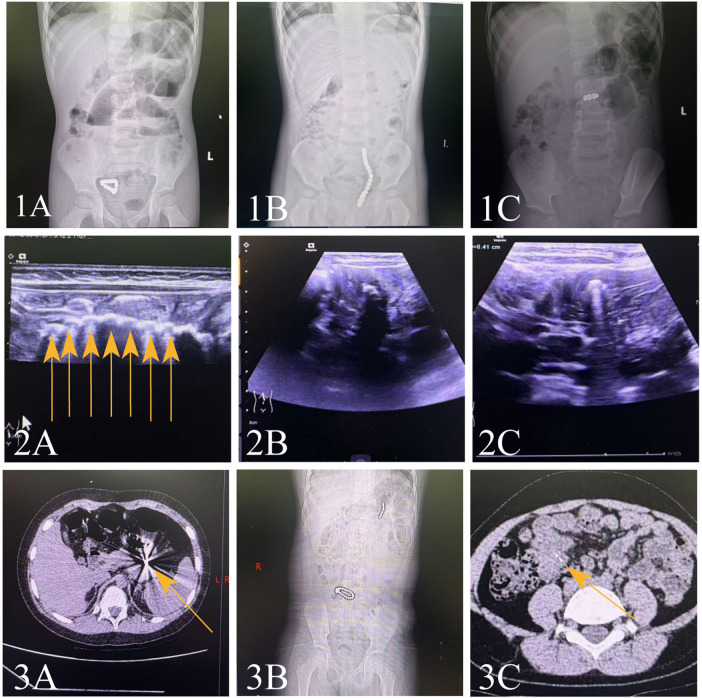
Using different imaging modalities to evaluate the preoperative location and condition of magnetic beads within the abdominal cavity. (1A–1C) show upright abdominal radiographs demonstrating clearly visible magnetic beads distributed within the gastrointestinal tract, presenting as “bracelet-like” and “string-of-beads–like” configurations. (2A–2C) show the ultrasound findings of one child. Spherical hyperechoic foci with posterior acoustic shadowing are visible within the gastric lumen. More than ten bead-like hyperechoic foci are arranged in a row within the mid-upper abdominal bowel loops, accompanied by focal bowel wall thickening (yellow arrow). A whirlpool-like torsion is observed at the root of the upper abdominal mesentery. (3A,3B) show CT findings of another child. In (3A), hyperdense foreign bodies are visible within the lower abdominal bowel loops, accompanied by marked metal artifacts (yellow arrow). (3B), an X-ray image reconstructed from CT, demonstrates the distinct distribution of magnetic beads in varying quantities. (3C) shows CT findings of a third child, again revealing hyperdense foreign bodies within the lower abdominal bowel loops with prominent metal artifacts (yellow arrow).
